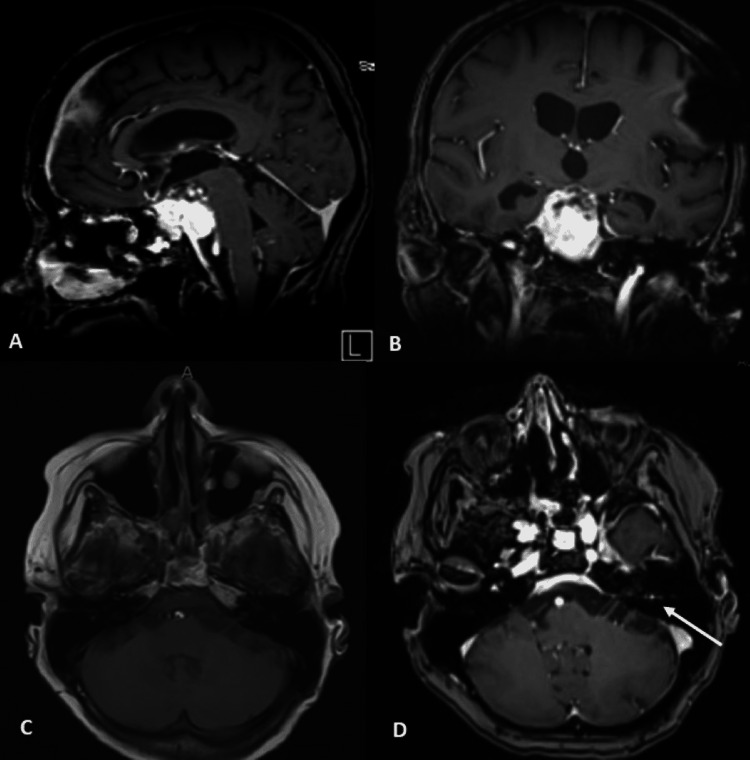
Top row: T1 post-contrast sagittal (A) and coronal (B) MRI images 2 months after initiation of the radiation therapy showed control and a slight decrease in solid tumor growth. Bottom row: T1 pre-contrast (C) and post-contrast (D) axial MRI. New enhancement was seen along the left facial nerve two months after initiation of radiation therapy concerning for leptomeningeal disease.
